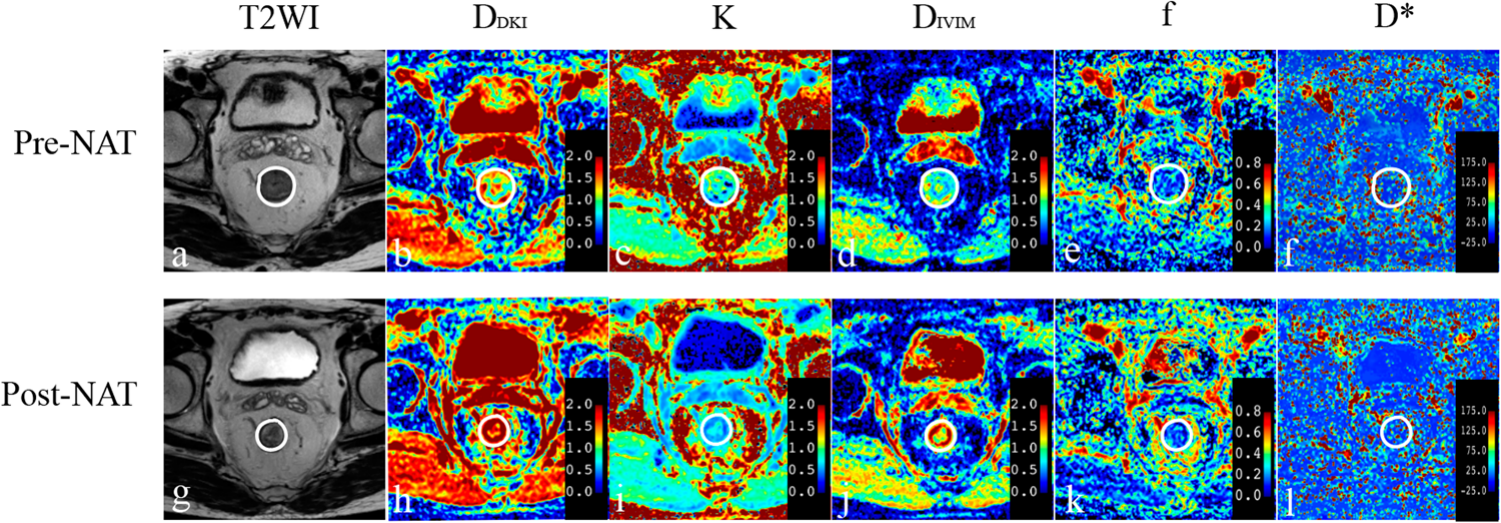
A 58-year-old man with pCR to NAT. Images in sequence are pre- and post-NAT MR imaging and IVIM–DKI parametric maps. Pre-NAT T2WI (a) and post-NAT T2WI (g) show an intermediate signal intensity rectal cancer at its largest dimension. The corresponding pre-NAT *D*_DKI_ map (b), pre-NAT *K* map (c), pre-NAT *D*_IVIM_ map (d), pre-NAT *f* map (e), pre-NAT *D*^*^ map (f), post-NAT *D*_DKI_ map (h), post-NAT *K* map (i), post-NAT *D*_IVIM_ map (j), post-NAT *f* map (k), and post-NAT *D*^*^ map (l) are shown. T2WI, T2-weighted imaging; *D*_DKI_, diffusivity of DKI; *K*, kurtosis of DKI; *D*_IVIM_, true diffusion coefficient; *f*, perfusion fraction; *D*^*^, pseudo-diffusion coefficient
